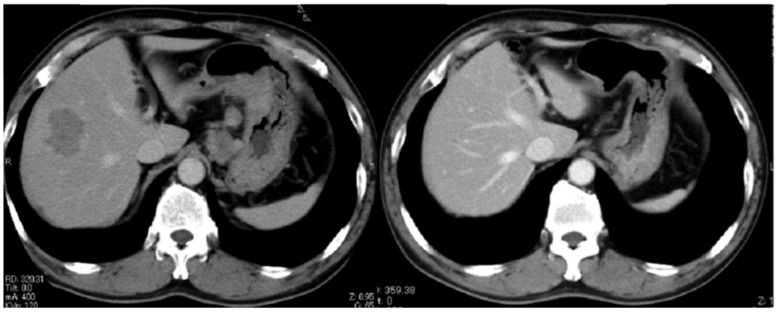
CT of Case 1, before (left; 02/08/’05) and after (right; 19/02/’09) the programmed chemotherapy. 60 yrs old male with melena was introduced to our hospital. He had differentiated-type MGC with liver metastasis and local lymph metastases. He was classified as level 1 and was treated with S-1 plus CDDP combination for 6 cools. CR was confirmed radiologically.
